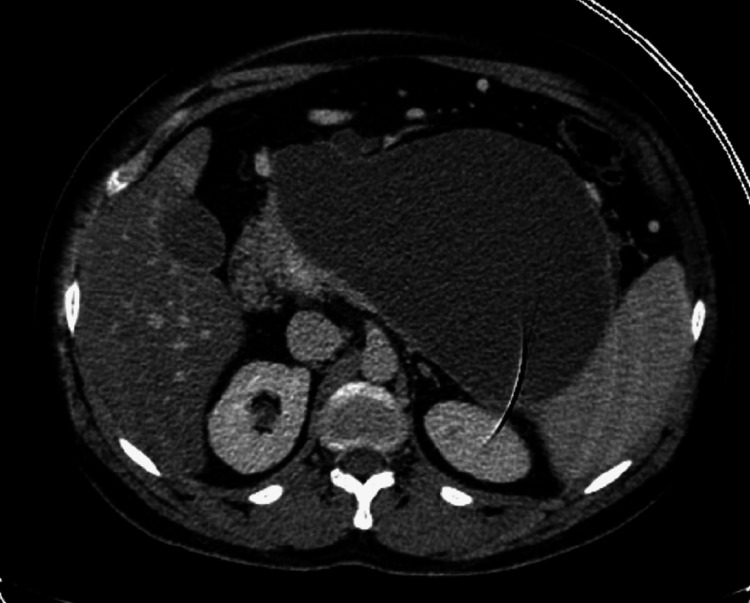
Axial section of CT scan showing pancreatic pseudocyst
